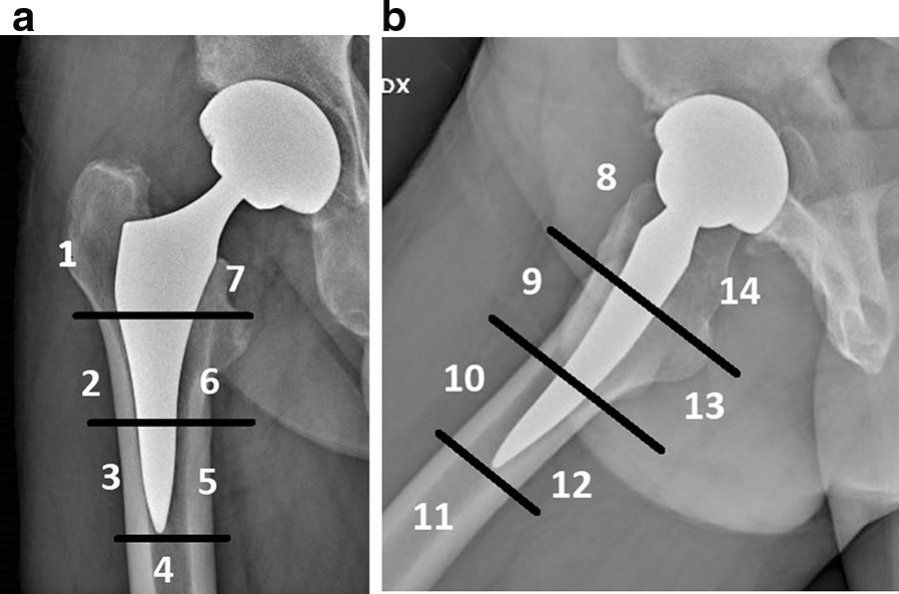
Radiographic follow-up and definition of Gruen’s periprosthetic zones in anteroposterior view (a) and axial view (b)
